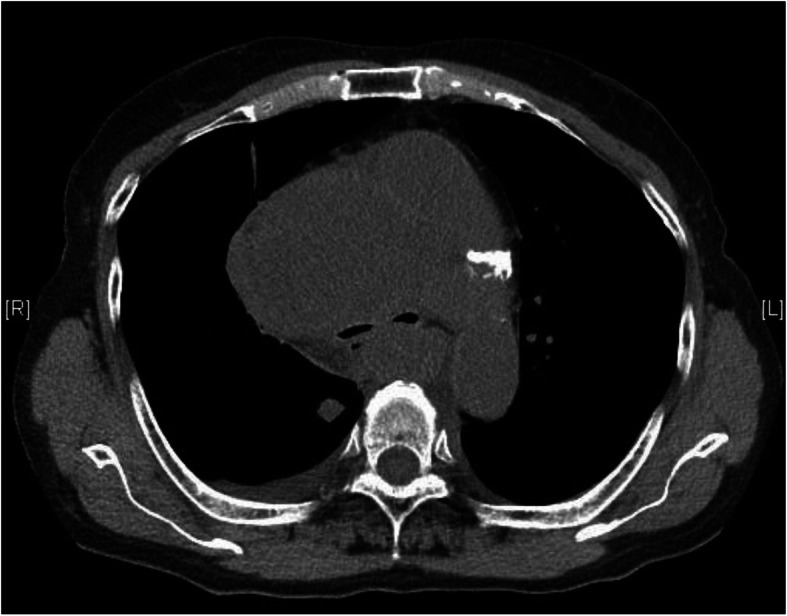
Preoperative chest computed tomography at the level of the main bronchus. Bilateral main bronchi were remarkably compressed by the anterior mediastinal tumor
